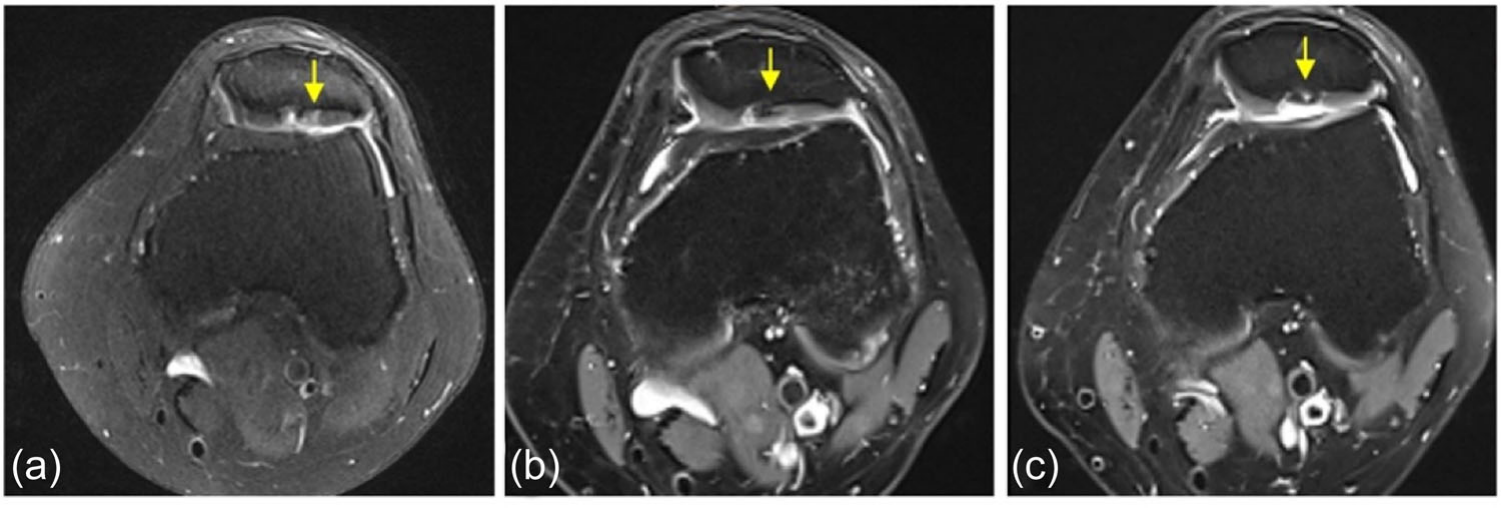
Example of poor tissue regeneration, the magnetic resonance imaging (MRI) of patient #12, left knee. (a) Preoperative MRI: Focal chondral lesion of the patella grade International Cartilage Regeneration Joint Preservation Society IV located in the central‐lateral facet (arrow). (b) MRI at 2 years: Decrease of the new tissue thickness, no significant signs of delamination, presence of a subchondral osteophyte, smooth interface edges and a small subcortical cyst in the plate (arrow). (c) MRI at 5 years: No changes from the previous MRI except for subchondral osteophyte growth (arrow).
